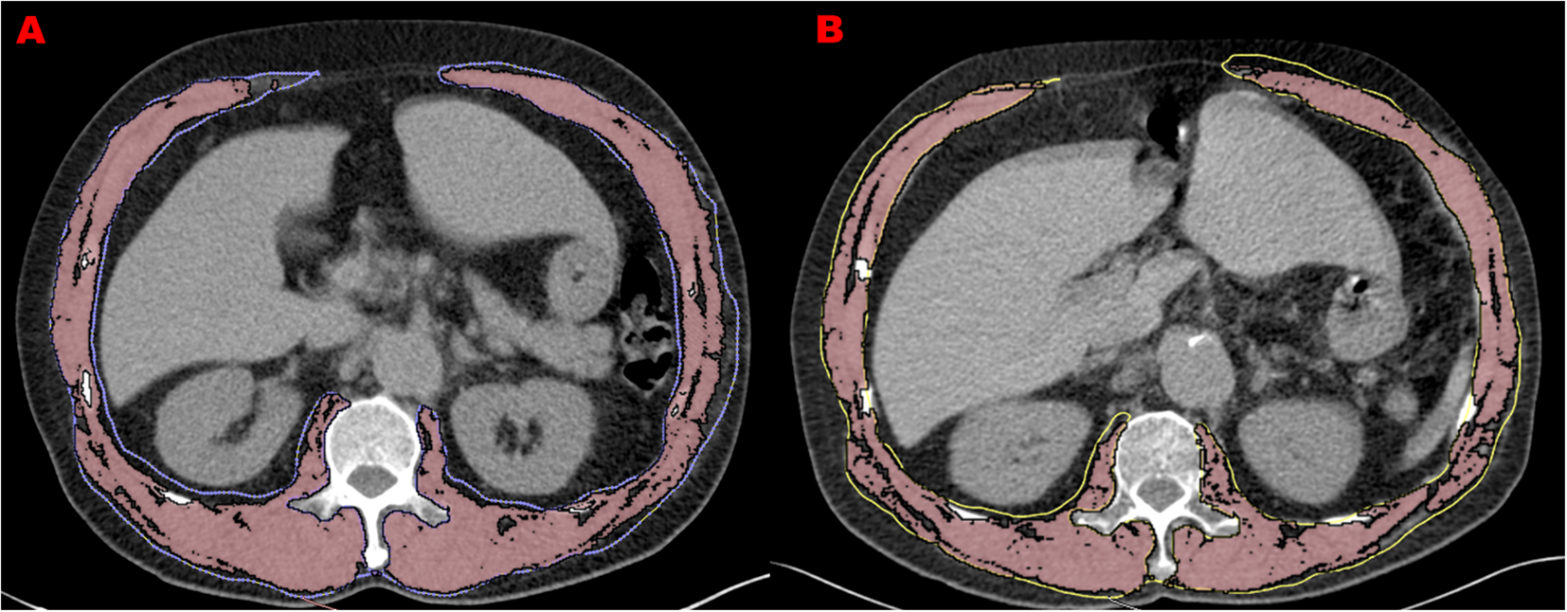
Muscle measurements at two screening chest CTs in 66-year-old man with COPD. (A) refers to L1 level CT image of 1^st^ CT scan with the measurement of trunk muscle density (31.9 HU) and size (140.7 cm^2^). (B) refers to L1 level CT image of 2^nd^ CT scan performed 3 years after the initial one with the measurement of trunk muscle density (26.1 HU) and size (114.8 cm^2^).
